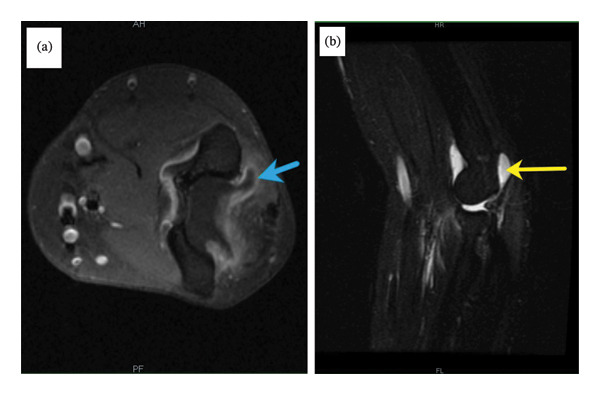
Magnetic resonance imaging of the left elbow demonstrated joint effusion with synovial enhancement on (a) T1‐weighted post gadolinium axial view (blue arrow) and (b) T2‐weighted post gadolinium sagittal view (yellow arrow). Arthrocentesis of the effusion provided the synovial fluid for analysis.
